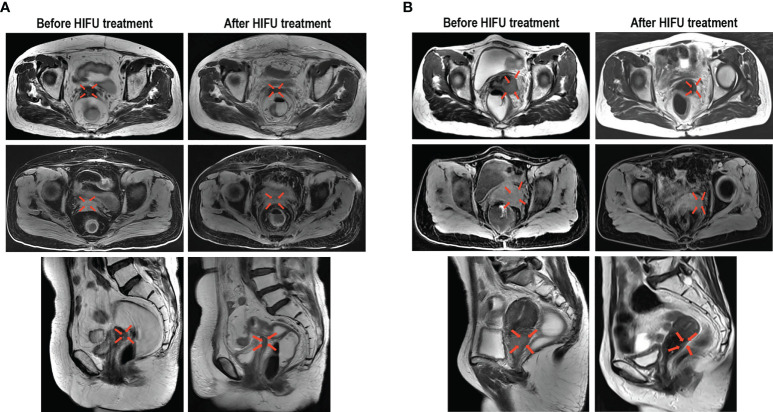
Representative images demonstrating the reduction of cervical cancer lesions following HIFU treatment. (A) Images from a 54-year-old patient with stage IIB cervical cancer before and after HIFU treatment, which display residual lesions that were previously treated with CRT. (B) Images from a 55-year-old patient with stage IIIB cervical cancer before and after HIFU treatment, which display recurrent lesions that occurred within an area that was previously irradiated. The transverse T2 MR images, transverse T1-fs MR images and sagittal T2 MR images all show that the tumor was significantly reduced.
